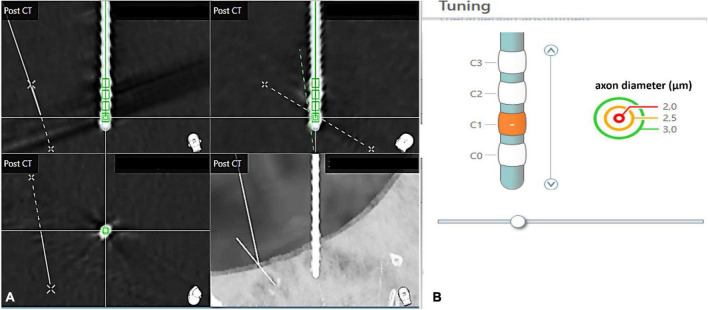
Methodological approach of electrode localization within the SureTune™ software. After definition of the AC-PC line in the axial and sagittal plane in the merged CT and MRI images, determination of spatial parameters of electrode localization was performed (A). Definition of active contact and modeling of volume of tissue activated by using corresponding stimulation parameters (B).
